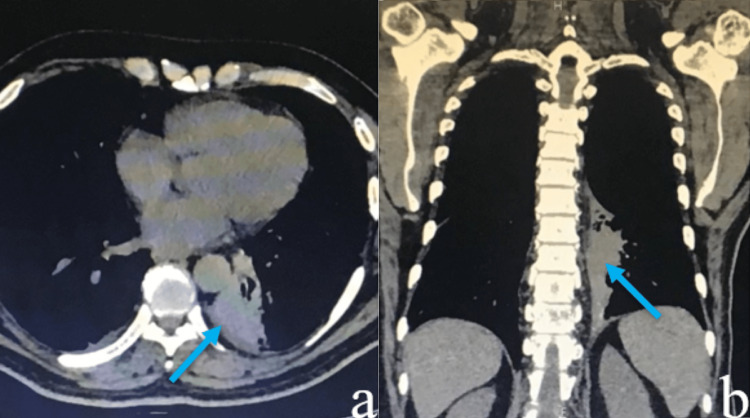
CT demonstrating the left hilum involving the left lung bronchus (a), with the destruction of dorsal vertebrae (b).
